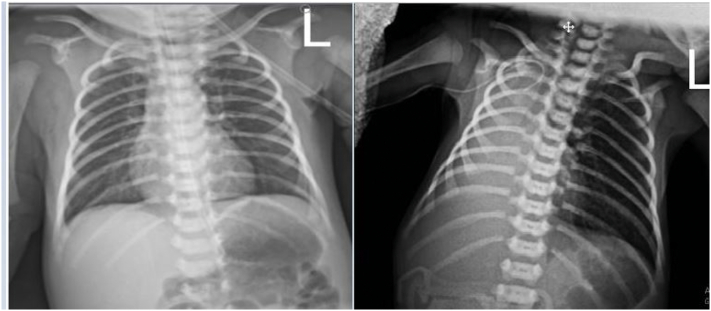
Radiograph of the newborn baby's chest before (left) and after (right) the PICC placement.
